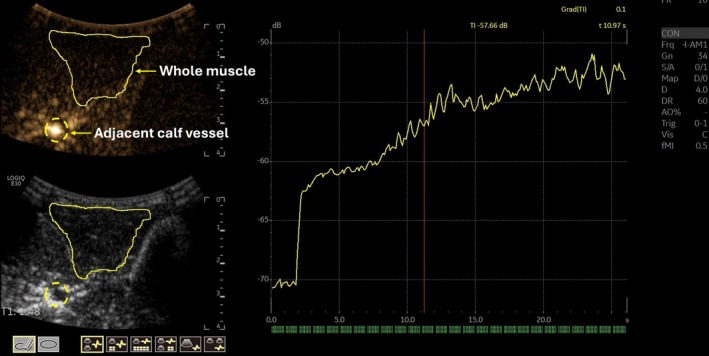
An example of SHAPE power optimization. After peak enhancement is achieved, the SHAPE optimization algorithm is initiated. The SHAPE optimization acquires subharmonic imaging from mechanical indices starting from 0.0 to 0.5 over a 26 s timeframe. This increase in acoustic output from a gradual increase from MI of 0.0 to 0.5 over 26 s generates a time intensity curve. The optimal power corresponds to the steepest slope, as this is where the subharmonic signal is most sensitive to the ultrasound contrast agents for pressure estimation. The only anatomical structure that was used to determine the power optimization was the whole muscle (solid yellow region of interest). The adjacent calf vessel (yellow dotted circle) is not included in the power optimization but is added as an example of the gradient used for SHAPE.
